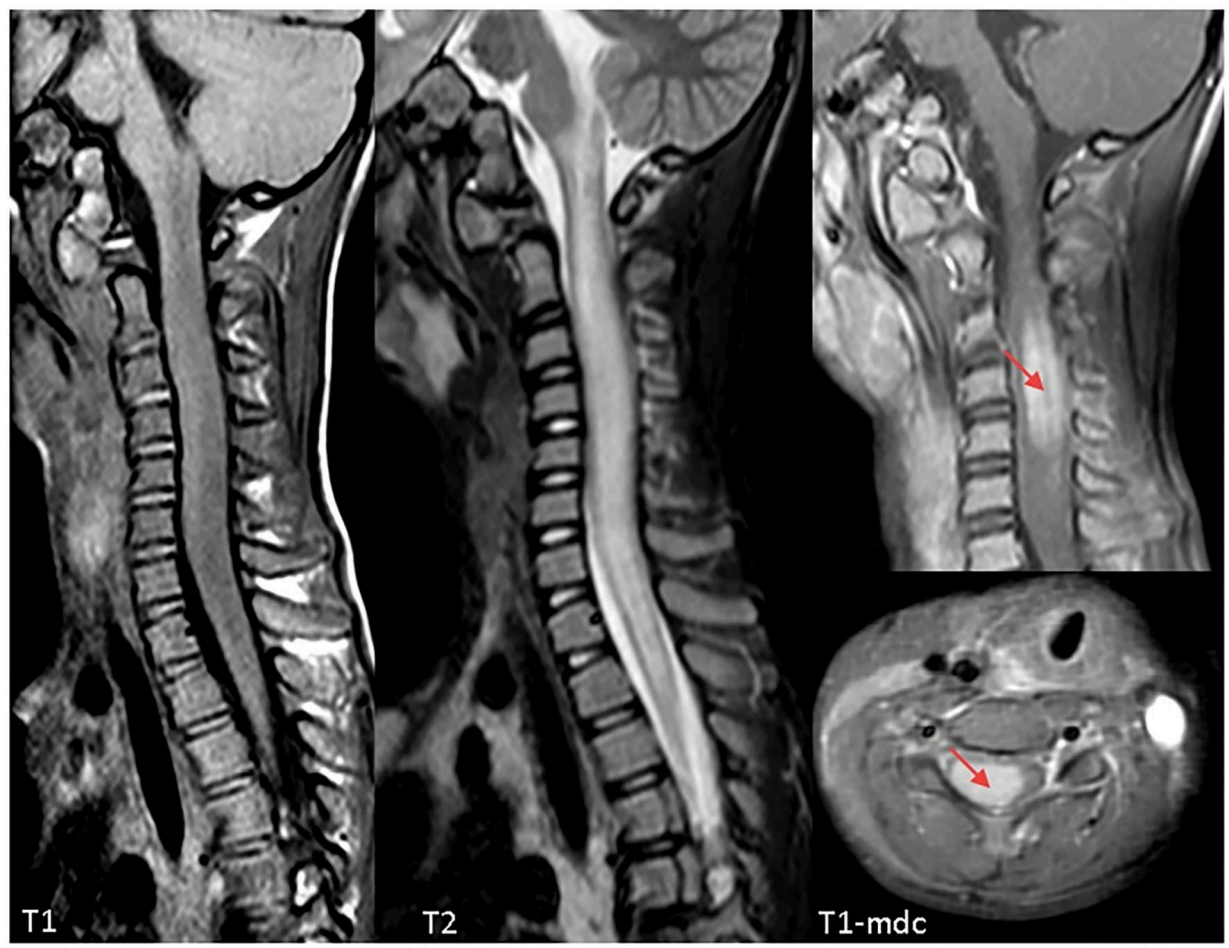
Cervical Magnetic Resonance Imaging (MRI) at clinical onset demonstrating a longitudinally extensive signal abnormality involving the cervical spinal cord, extending from the obex to D3. The cord appears markedly swollen, both gray and white matter are involved, and post-contrast sequences demonstrate marked cord enhancement between C3 and C5 (red arrows). Findings are compatible with acute longitudinally extensive transverse myelitis (LETM).
